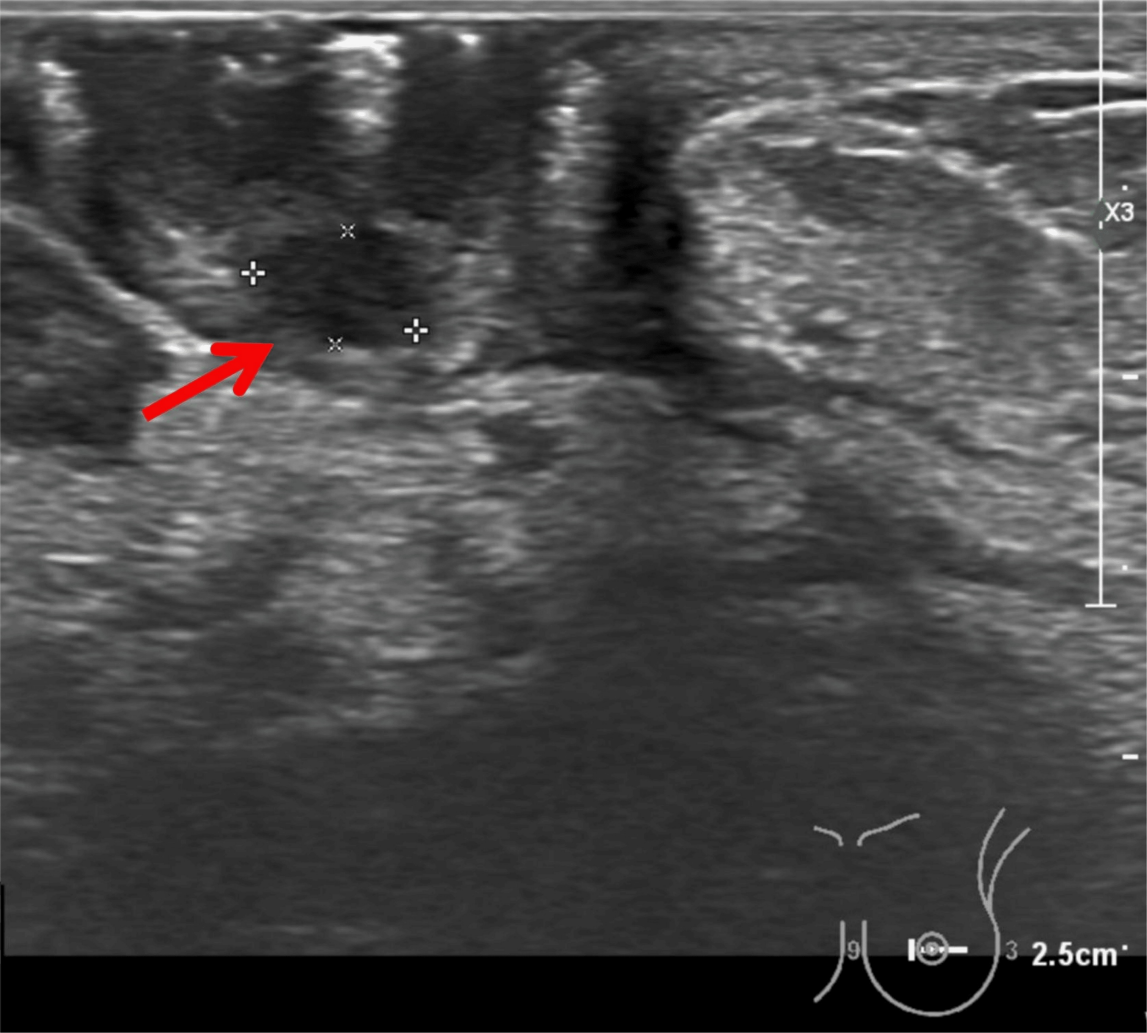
Ultrasonographic Findings of the Left Breast (A) Grayscale ultrasound image showing a well-defined hypoechoic nodule located deep to the left nipple (red arrow). The lesion measures approximately 4.6 mm × 3.0 mm, appears oval in shape, and exhibits no significant posterior acoustic enhancement or shadowing.
